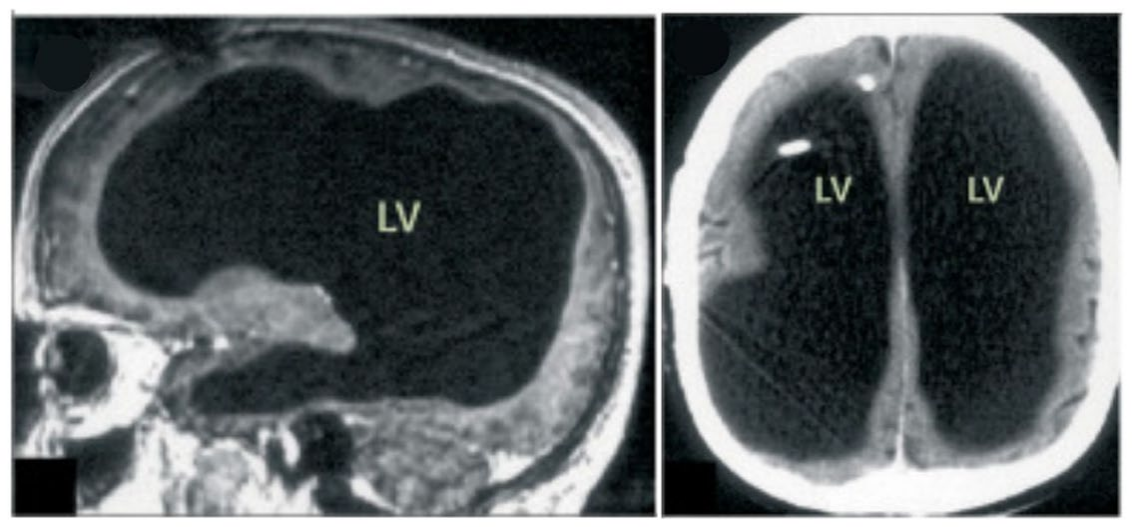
MRI image of a hydrocephalus brain. Credit: Feuillet et al. (2007). Copyright 2022, reproduced with permission from Elsevier.
